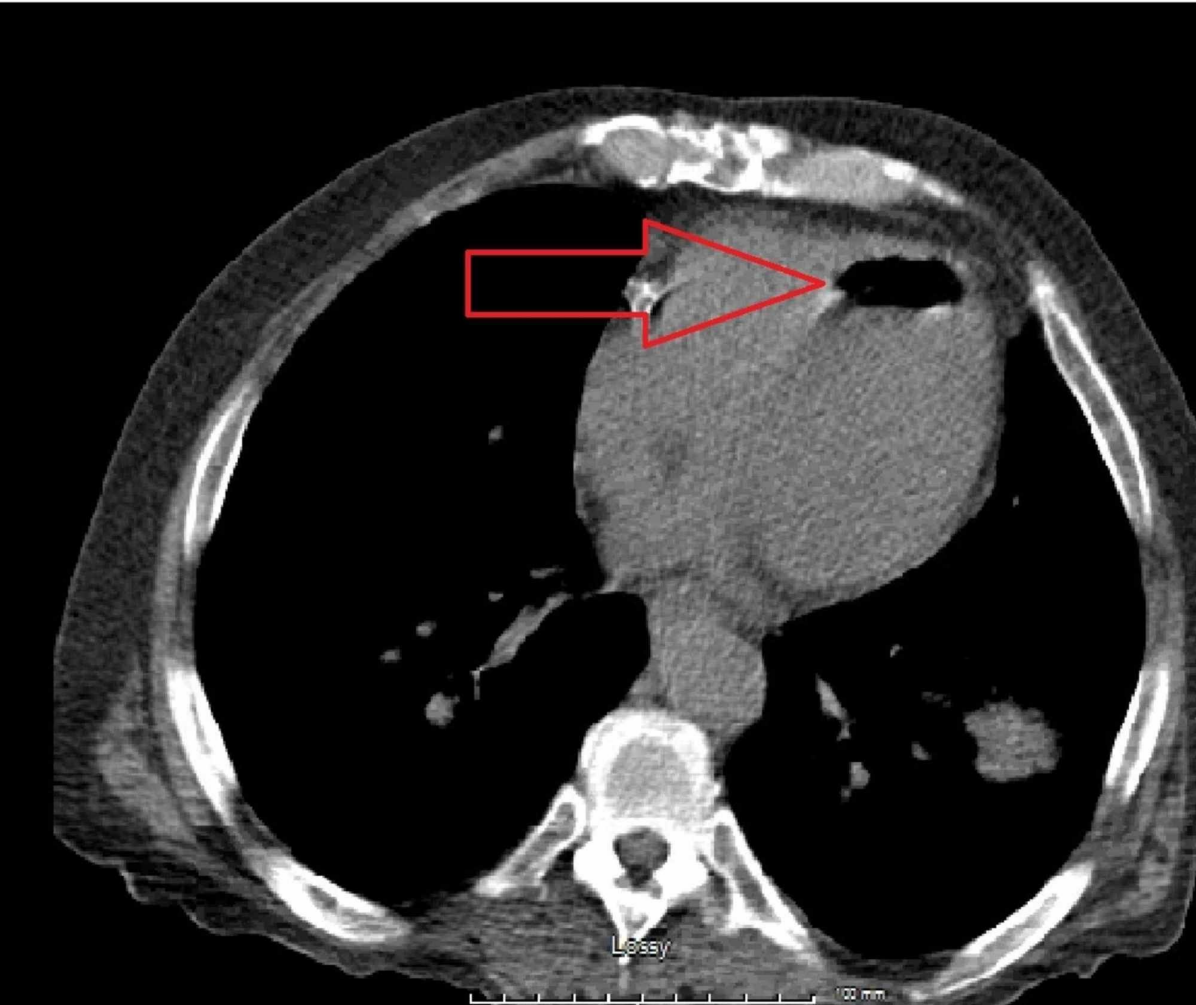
Computed tomography (CT) scan of chest showing air embolism in left ventricle
